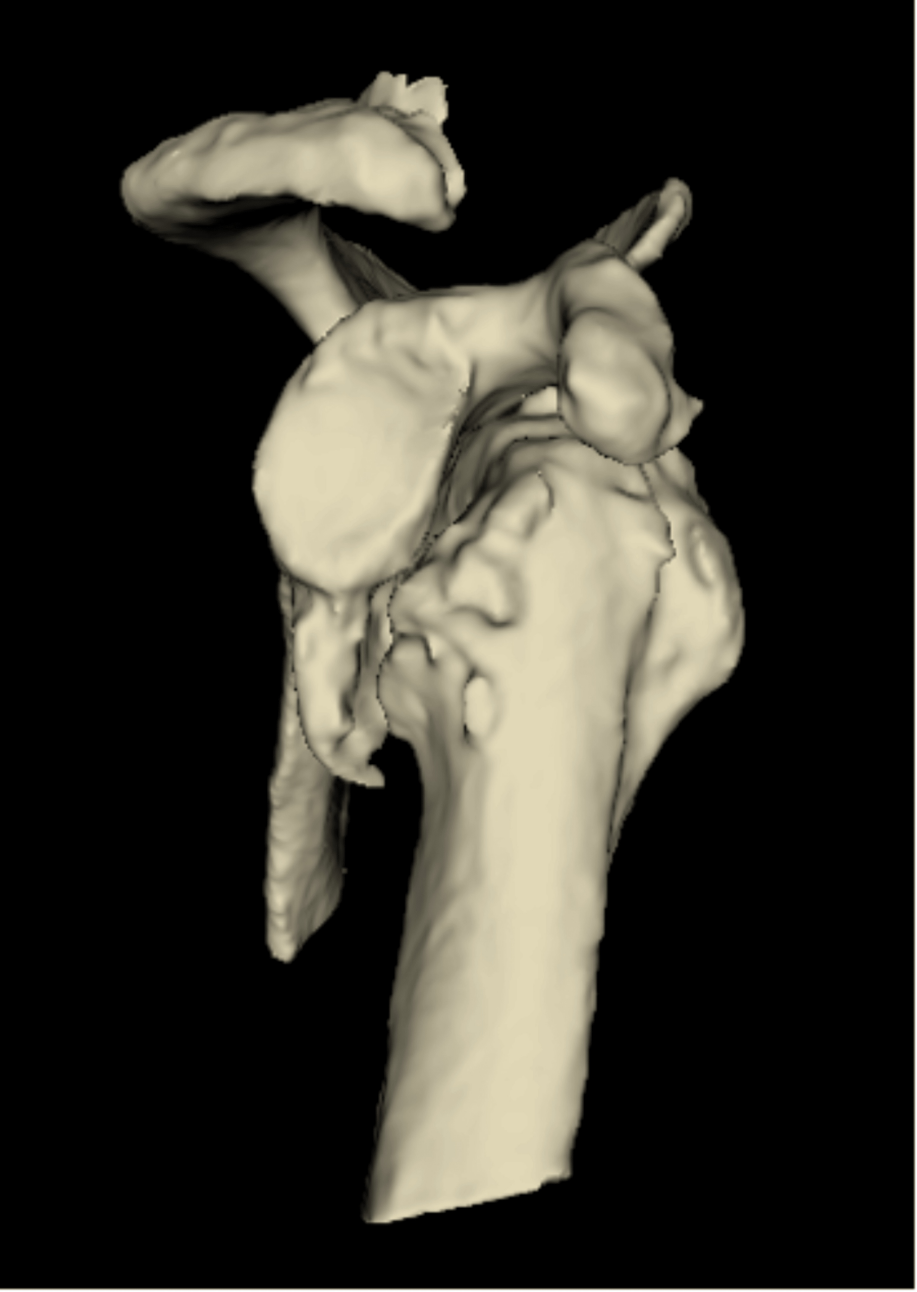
Pre-operative 3D CT sagittal view of the glenohumeral joint demonstrating anterior shoulder dislocation and a glenoid bony defect.
